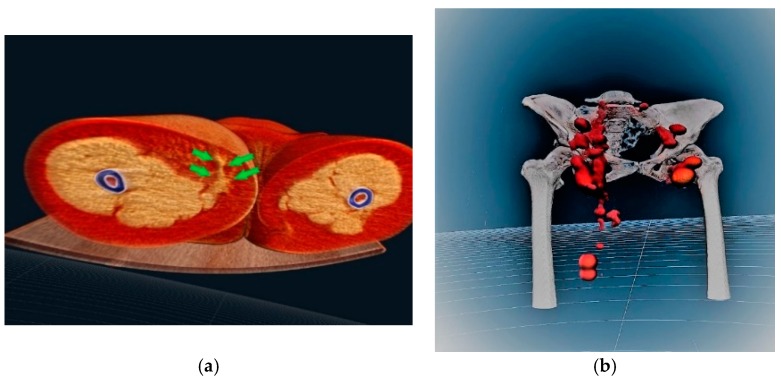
(a) 3D constructed model based on the fusion of the radiographic images and obtained by virtual reality software showing the lymphatic malformation (green arrows) (b) 3D reconstructed hybrid image of the SPECT-CT and lymphoscintigraphy obtained by virtual reality software: visualization of the lymphatic structures of the lower limbs. Note the extension of the malformation (conglomerate of tracer) from the region of the right thigh up to the abdominal cavity.
